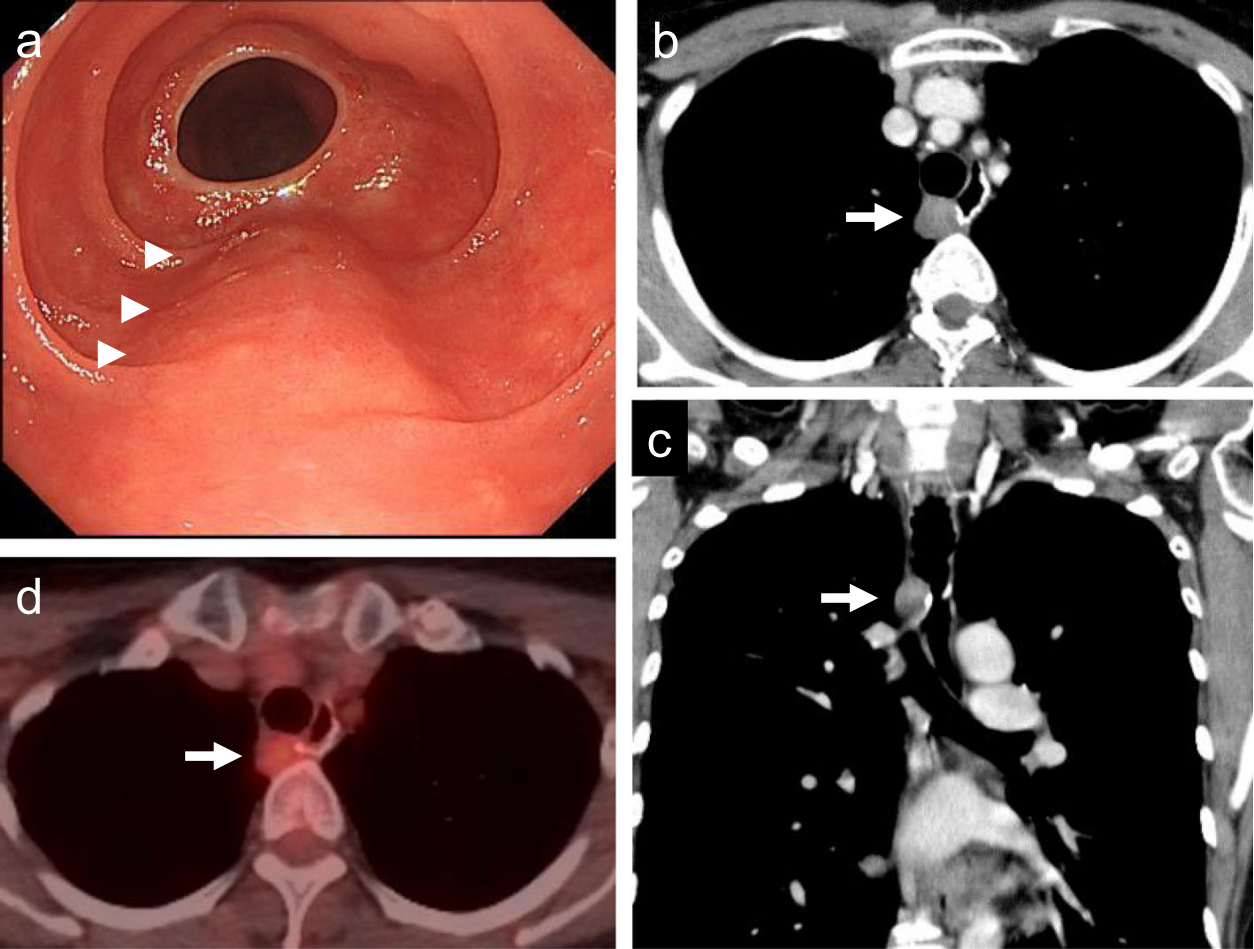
Images obtained before the third operation. Esophagoscopy showed a 20-mm-sized extrinsic mass located at the distal anastomotic site between the jejunum and the esophagus (arrowheads) (a). Contrast-enhanced CT showed a globular mass at the distal anastomotic site between the jejunum and the esophagus (arrow) (b, c). FDG-PET/CT revealed some FDG uptake in the tumor (arrow) (d)
